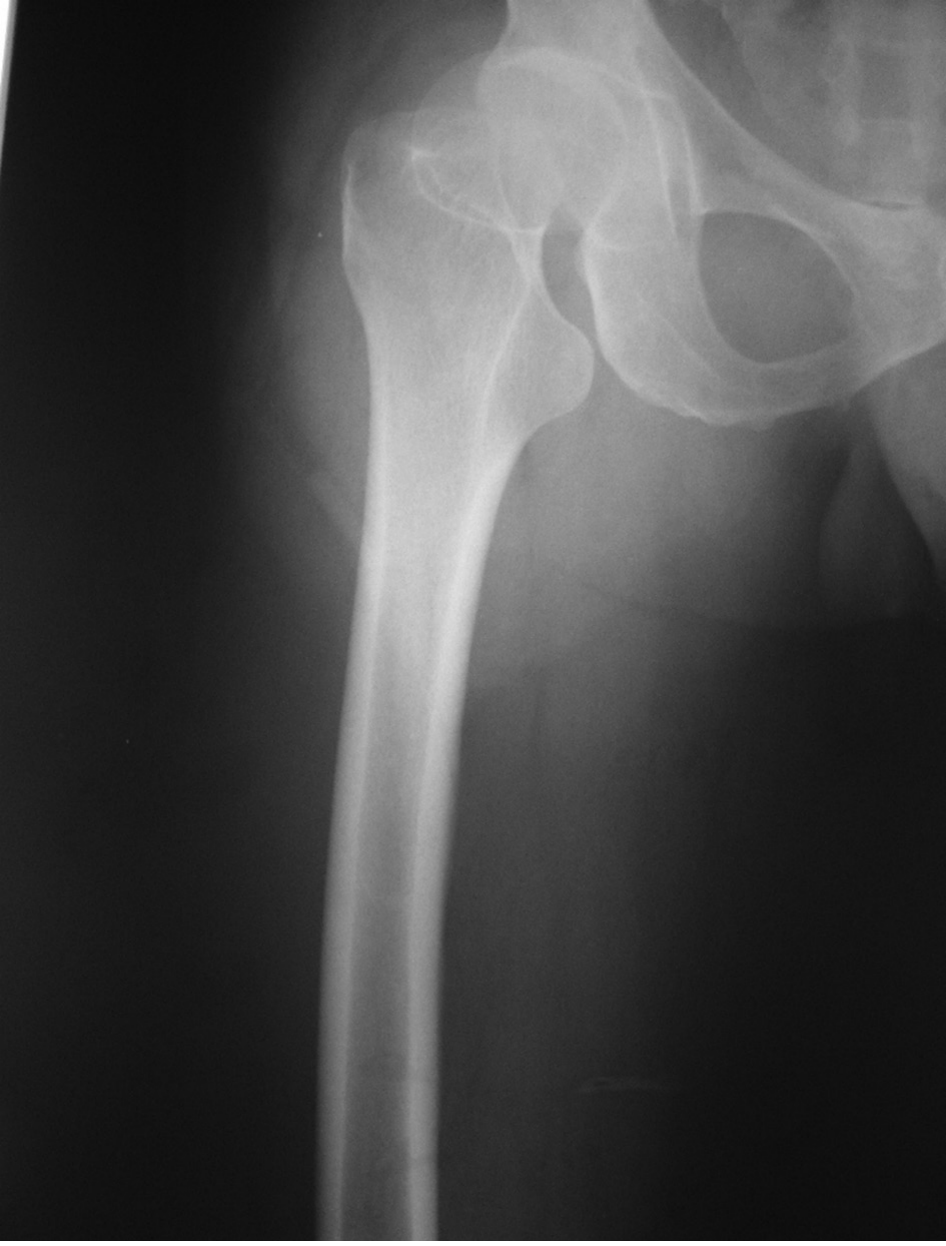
Anterior-Posterior X-ray Images of the Right Hip
